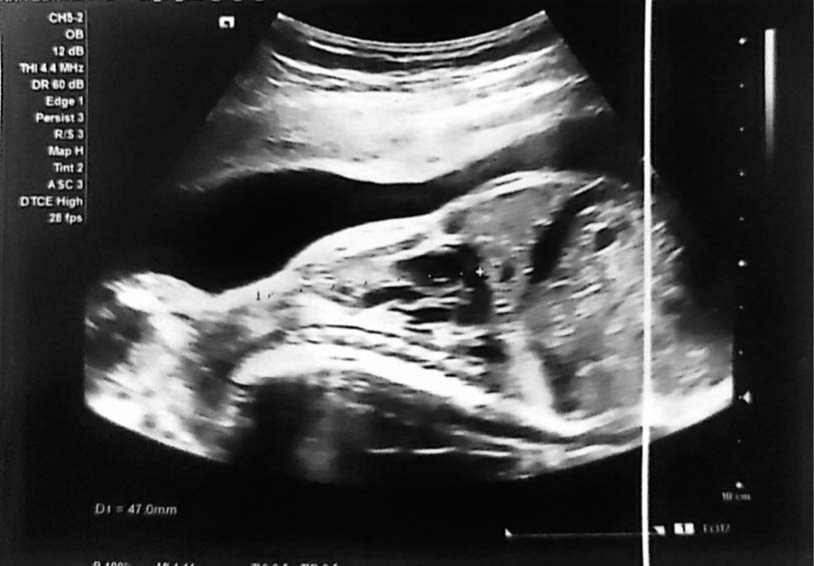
Ultrasonographic result of uterus showing clover-shaped skull, narrowed chest with short horizontal ribs, shortening of all the limbs.
